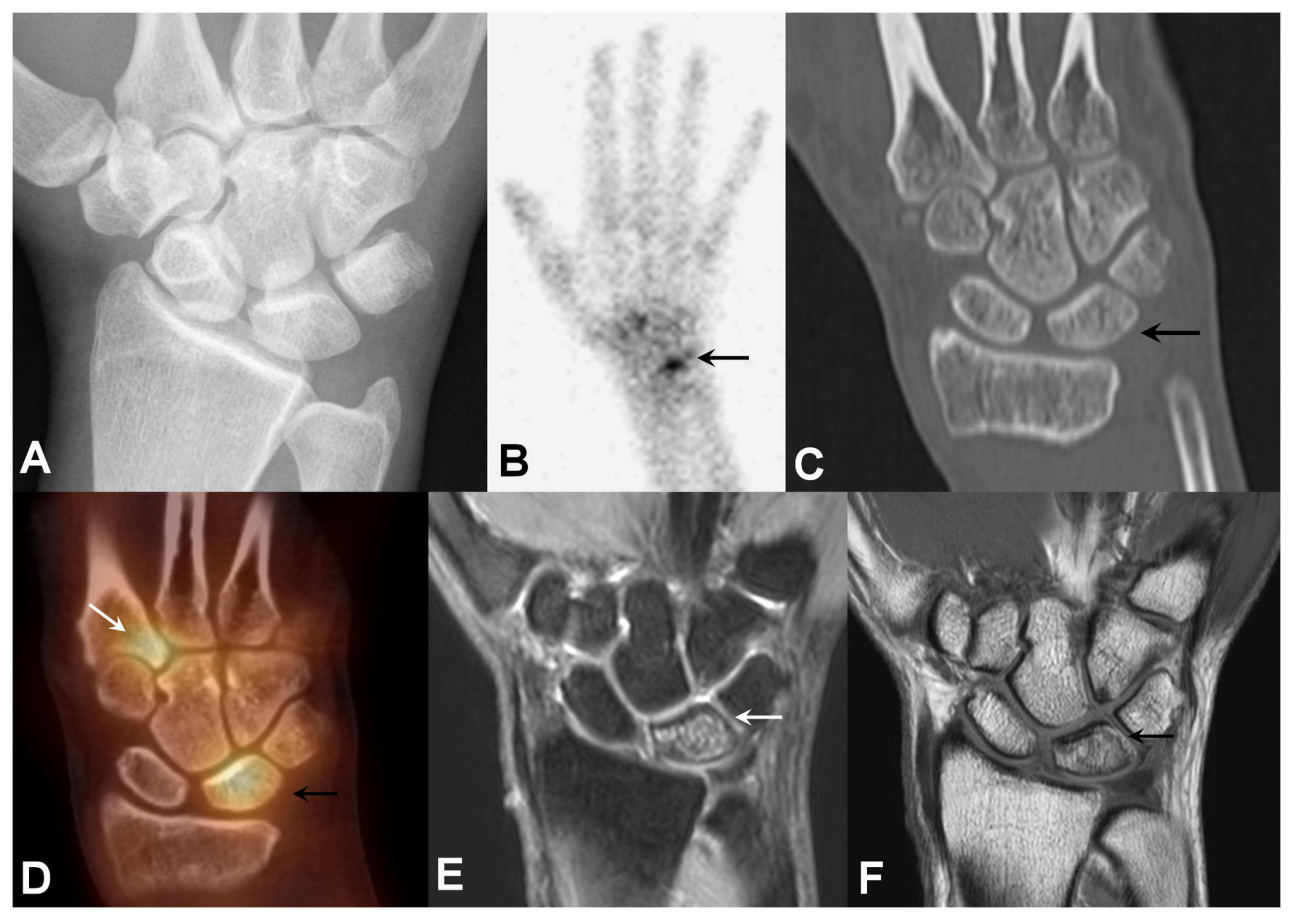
44-year-old female with osteomalacia of the lunate bone. Relative shortness of the distal articular surface of the ulna on plain radiograph (a), focally increased radiotracer uptake in the carpal region on bone scan (b), slightly hyperdense and coarse trabecular structure of the lunate bone on CT (c), tracer accumulation throughout the lunate bone on SPECT/CT fusion image (d), altered signal of lunate bone marrow in PDw SPIR (e) and T1w image (f) on MRI. Besides, there is also focal tracer accumulation in the ulnar-sided base of the 2^nd^ metacarpal (d) indicating a “carpal boss”, which was not in the main clinical focus at that time.
